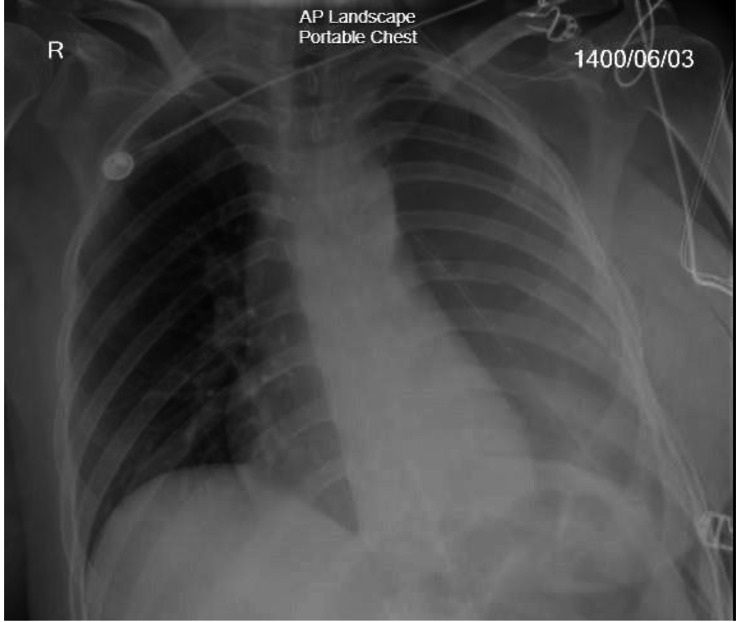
Post-operative chest radiography of a 39-year-old female patient with a diagnosis of pleural epithelioid hemangioendothelioma following extrapleural pneumonectomy
